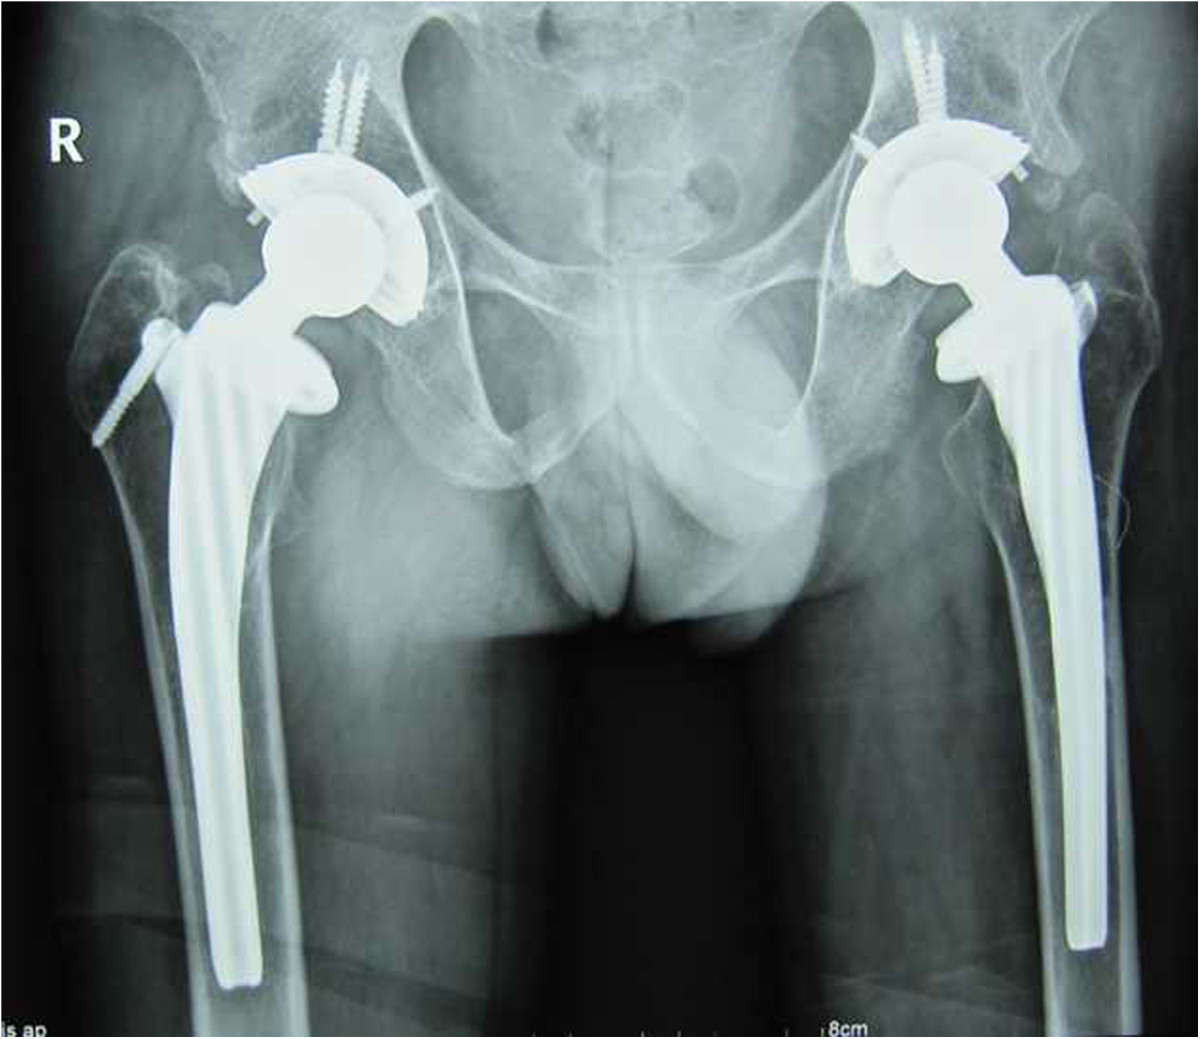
Postoperative radiograph of the patient 7 years after cementless THA.
